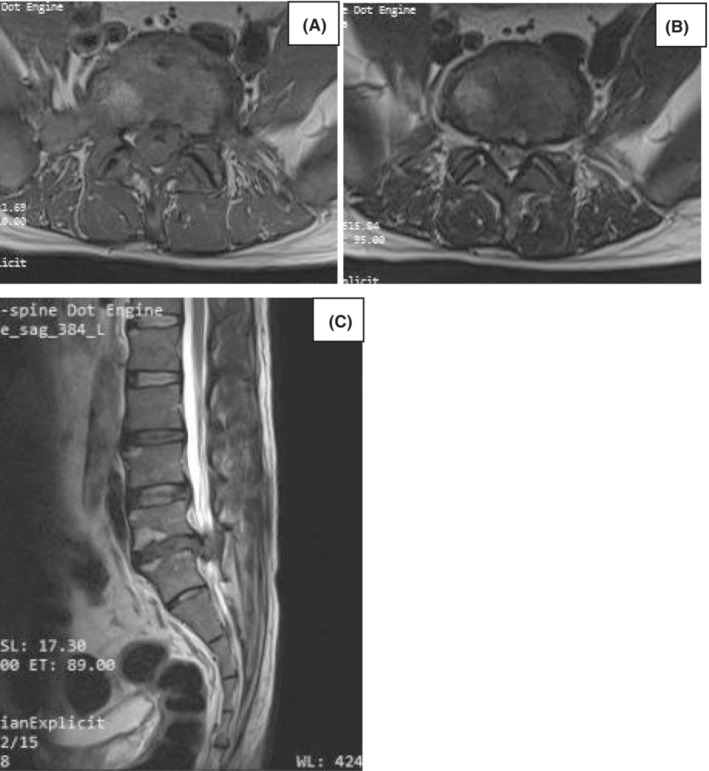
MRI lumbar T1 & T2 axial and T2 sagittal revealed focal posterocentral disc bulge with central and B/l paracentral extrusion of the disc elements in the superior and inferior spinal canal at L5‐S1 level causing severe narrowing of the central spinal canal and compression of the descending nerve roots as well as traversing S1 left nerve root.
